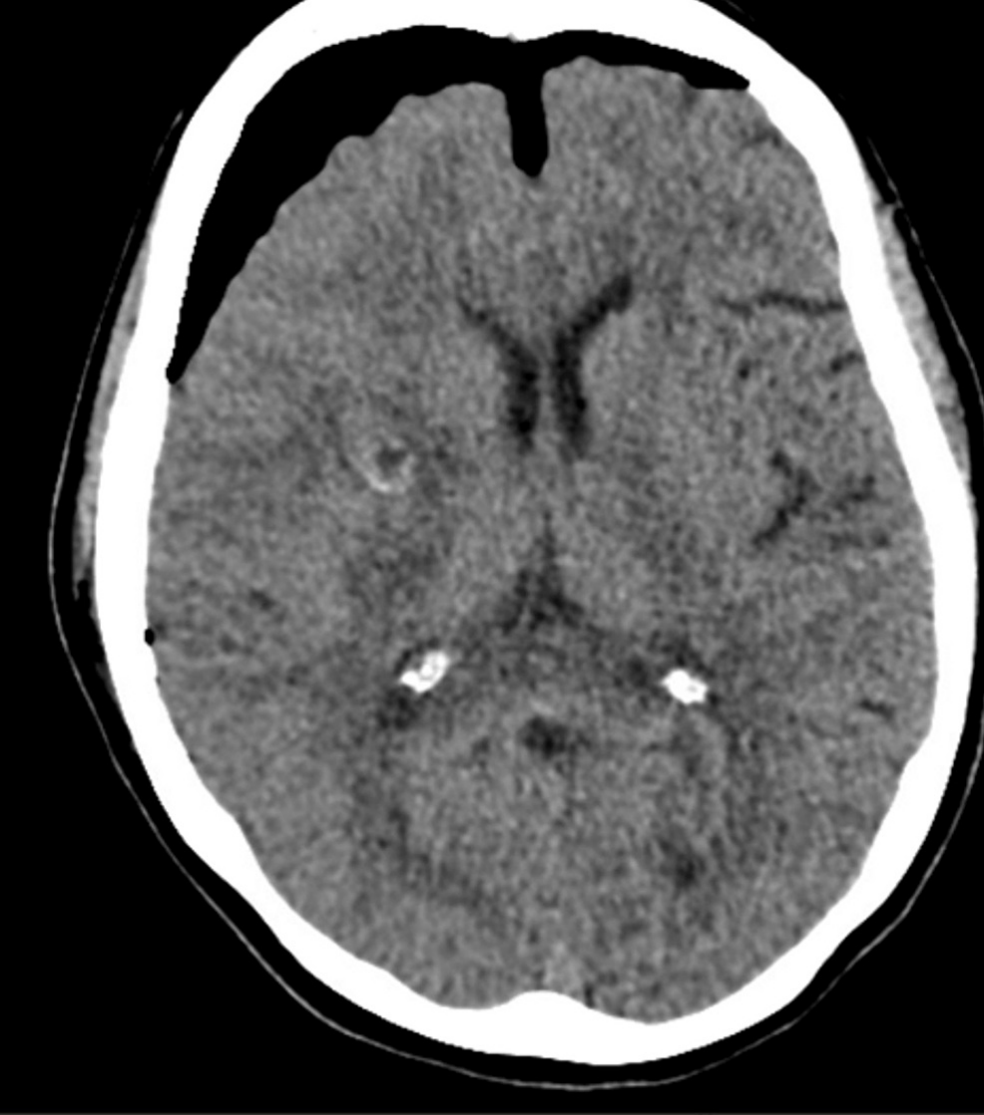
Post-op CT scan showing post-surgical changes and residual acute blood.
